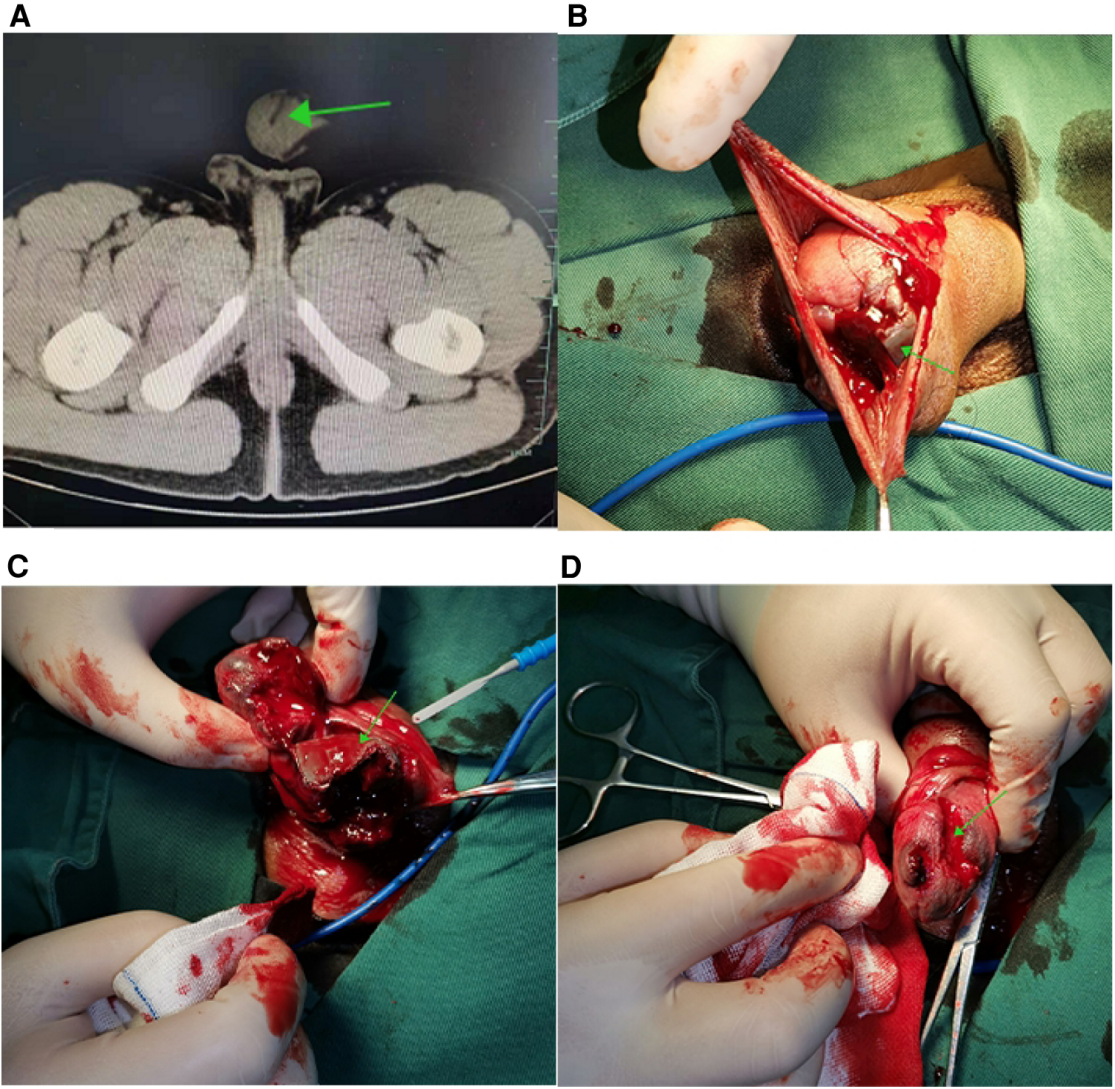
(A) CT scan demonstrating a foreign body penetrated the corpora cavernosum; (B) an irregular shrapnel penetrated from the balanus to the corpora cavernosum; (C,D) the shrapnel penetrated very deeply with active bleeding and removed the shrapnel carefully.
